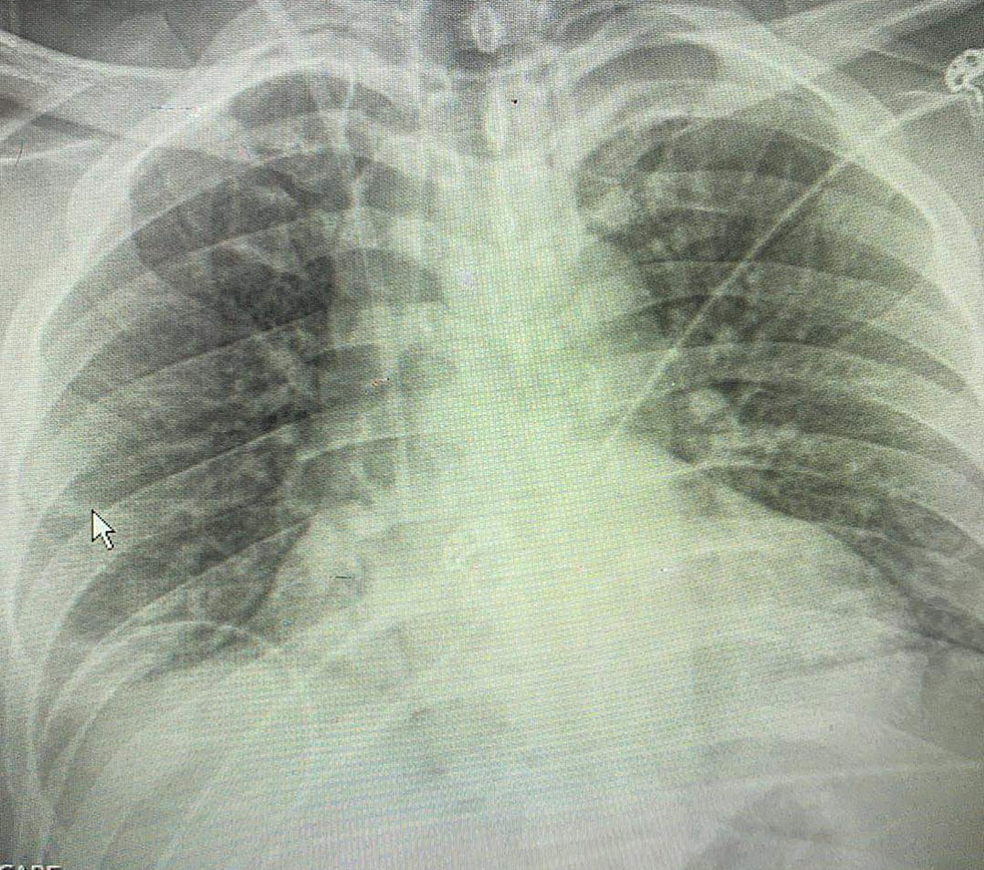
Chest x-ray showing the non-acute phase of COVID-19 pneumonia.
